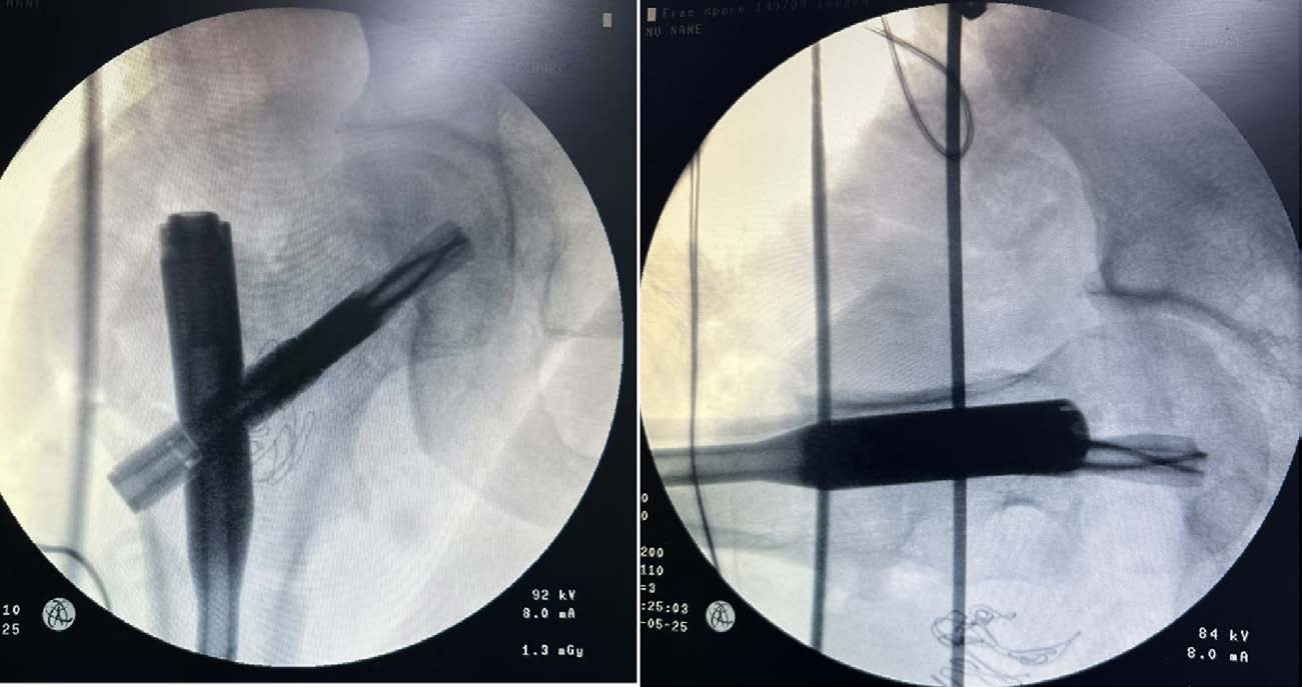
Intraoperative radiographic assessment of reduction and fixation for the intertrochanteric fracture.
